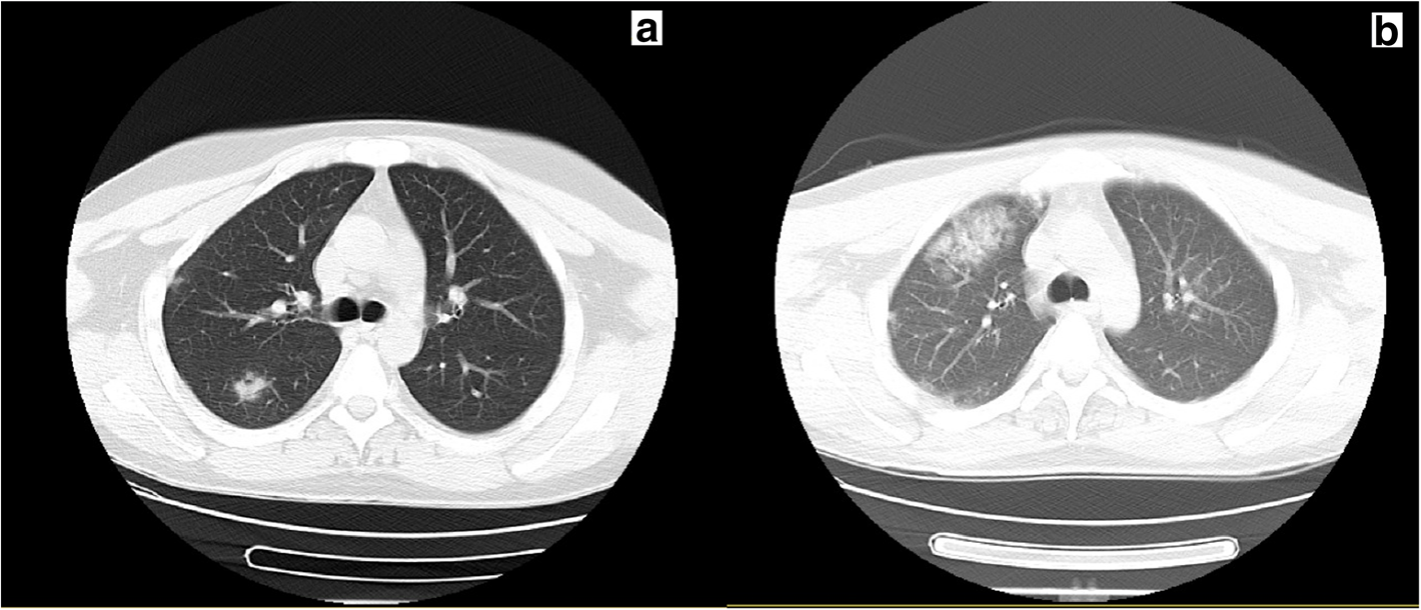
CT scan of the chest of the two identical twins with COVID-19. Figure “a” shows patient number 1 who previously had a liver transplantation and figure “b” shows patient number 2 who had no underlying disease. CT scan of patient “b” shows more severe pulmonary involvement by COVID-19
